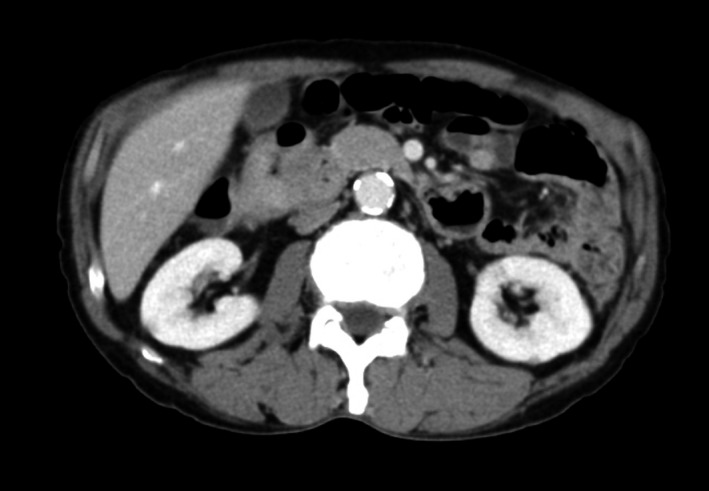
The computed tomography findings were highly indicative of duodenal and pancreatic head invasion of the transverse colon cancer
